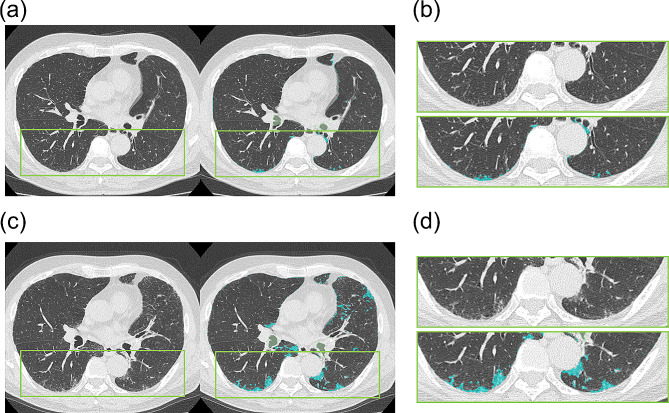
Representative images of a patient without ILA at enrollment who had ILA at follow-up Legend: Representative images are of a 77-year-old male patient who developed ILAs according to the follow-up CT scan. The patient did not have ILAs at baseline (shown in Fig. 4. a and Fig. 4. b), but some emerged at follow-up (shown in Fig. 4. c and Fig. 4. d). In the right panels of Fig. 4. a and Fig. 4. c and the lower panels of Fig. 4. b and Fig. 4. d, ILD regions segmented as GGOs, reticulations, and honeycombing using AIQCT are overlaid onto the CT images in light blue. The ILD volume was 47.3 ml (0.98%) at baseline and increased to 164 ml (3.4%) at follow-up
